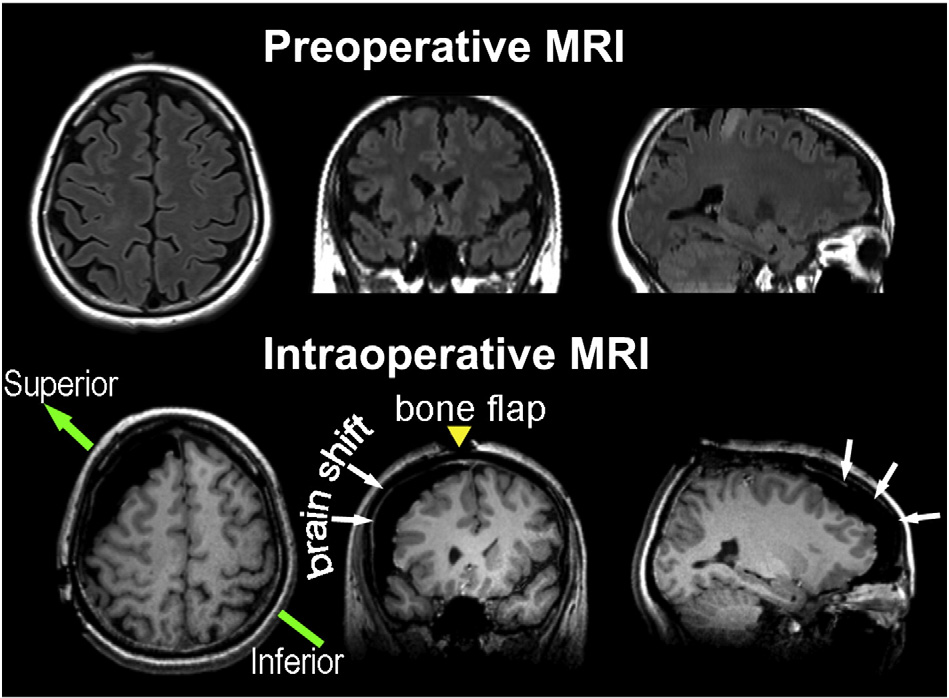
Demonstration of brain shift caused by craniotomy.* The brain shift was shown using the example of patient 7. The upper row shows the orthographic views of the preoperative structural image (T2-FLAIR). The lower row shows the corresponding planes of the same patient's intraoperatively acquired images (3D-T1-MPRAGE). White arrows show the brain deformation. The yellow arrow indicates the incision line on the scalp (bone flap). The green arrow indicates the direction of the patient's head on the operating table.
